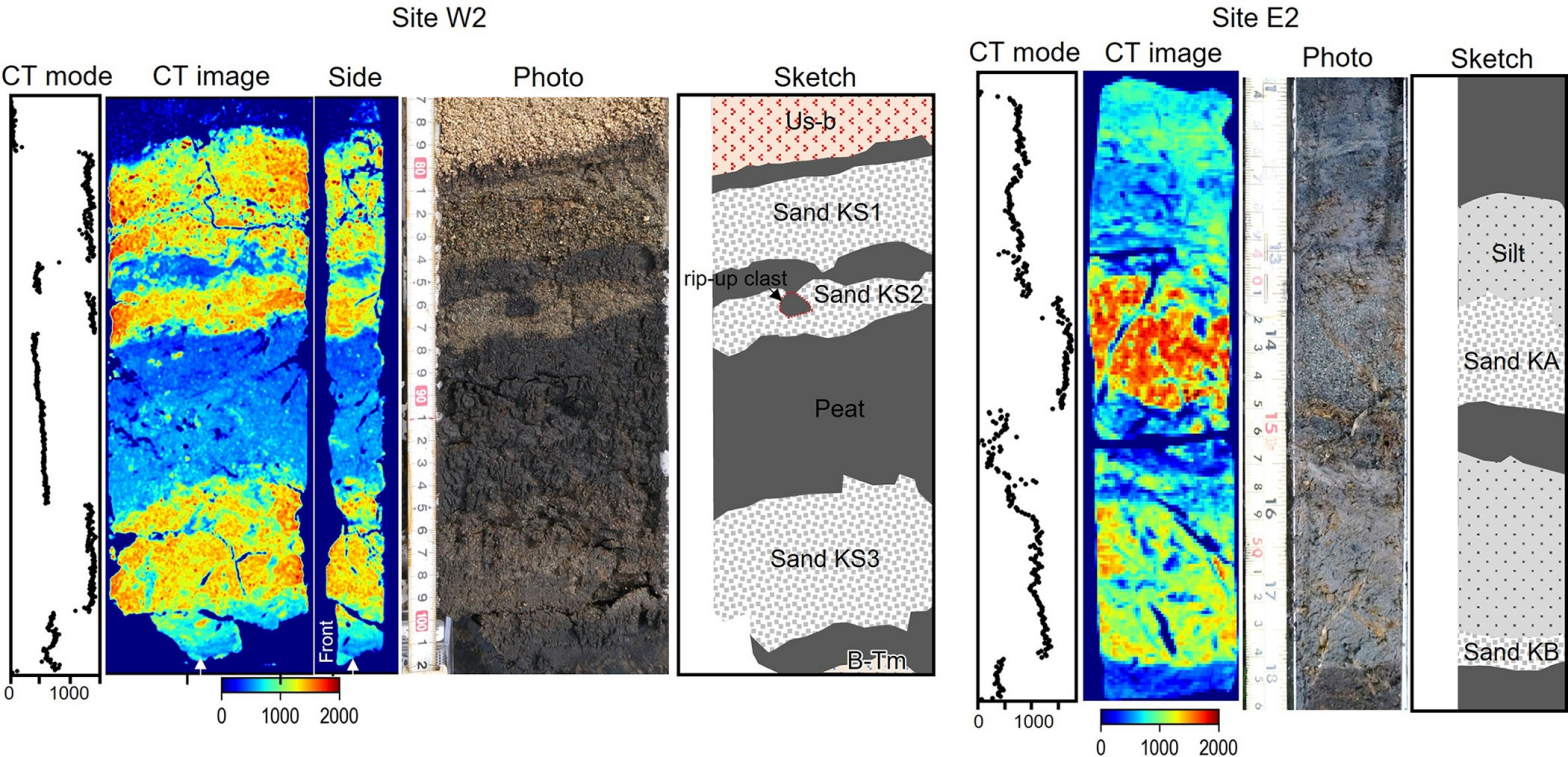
Core photographs and X-ray CT images at Sites W2 and E2. The CT mode profiles are plotted in correspondence with the CT images. For Site W2, a CT side image is shown, and its cross-sectional location is indicated by the arrows. The sketches display the interpretation of sedimentary structures of the sand layers and tephra layers.
